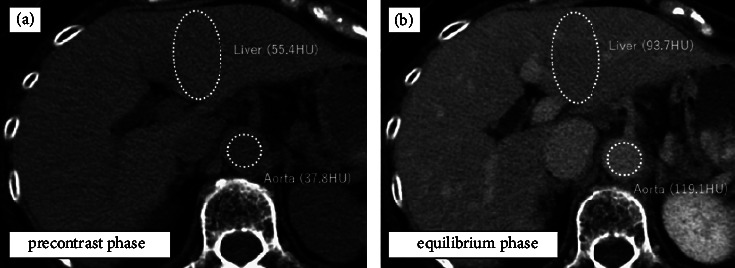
Preoperative computed tomography (CT) images from a 65-year-old woman with colorectal cancer liver metastasis after oxaliplatin-based chemotherapy. Oval regions of interest are placed and CT values are measured on the liver parenchyma and abdominal aorta in both precontrast (a) and equilibrium phase (b) images. On the basis of the hematocrit level (35.9%), ΔHU_liver_ (93.7 − 55.4 = 38.3 HU), and ΔHU_aorta_ (119.1 − 37.8 = 81.3 HU), the calculated extracellular volume fraction was (100 − 35.9) × 38.3/81.3 = 30.2%.
